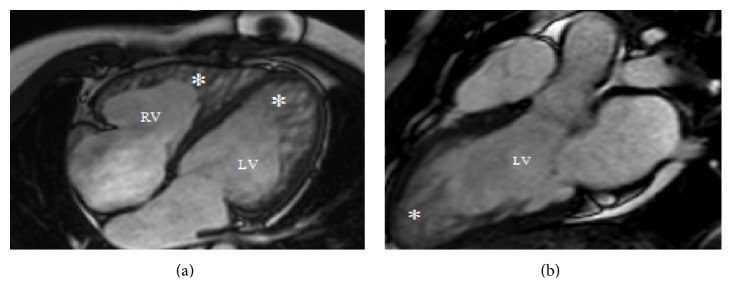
Cardiac MRI of the heart. (a) Cardiac MRI showing extensive biventricular trabeculations with (b) sagittal view demonstrating deep recesses in the left ventricle cavity (see asterisk).
